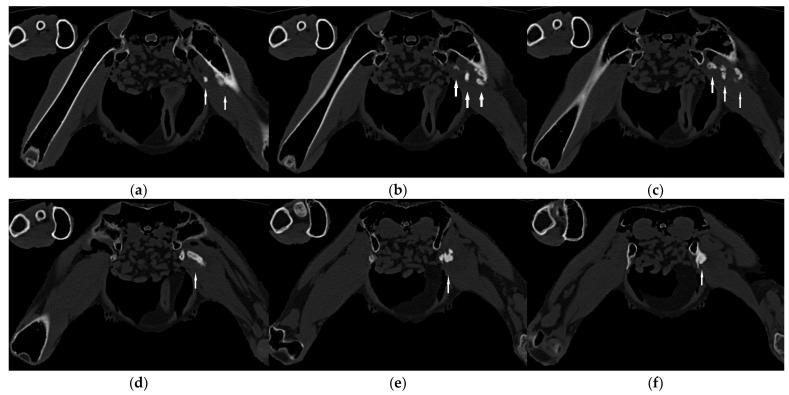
Axial computed tomographic images from the cranial (a) to caudal (f) area of the bald eagle of case 1 at the level of the hip joint (a–f). Heterotopic ossifications in the adductors (arrows) with a fusion in the pelvis (d) and the femur (a,b) and the partially existing eggshell appearance of the calcifications (b–d) are visible.
